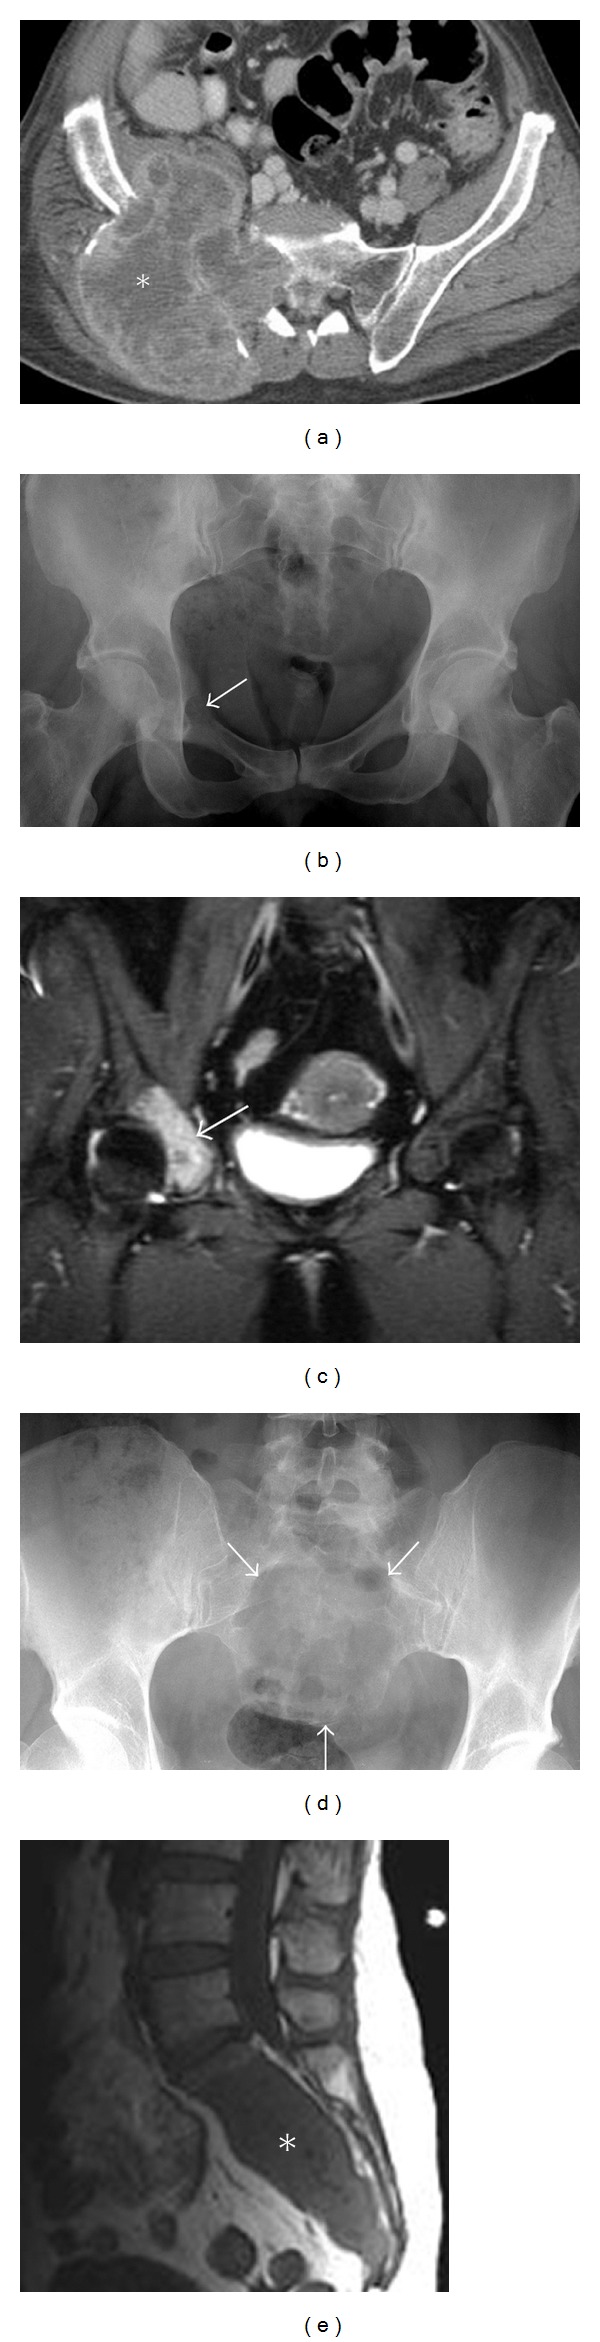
Metastasis. A 58-year-old male presents with a short history of right hip pain and a remote history of primary renal cell carcinoma diagnosed with metastases. Axial post-contrast CT of the pelvis (a) demonstrates a large destructive expansile necrotic mass (asterisk), arising within the right ilium and extending into the right sacrum. Two different patients with breast cancer metastasis to the acetabulum (b) and (c) and to the sacrum (d) and (e). These lytic lesions can be difficult to identify on plain films (b) and (d) more easily appreciated on coronal STIR (c) and sagittal T1 (e) MRI. Note the destruction of arcuate lines by the sacral lesion; destruction of the subarticular cortex, asymmetric density of the right acetabulum, and fuzziness of the right iliopectineal line by the acetabular metastasis.
